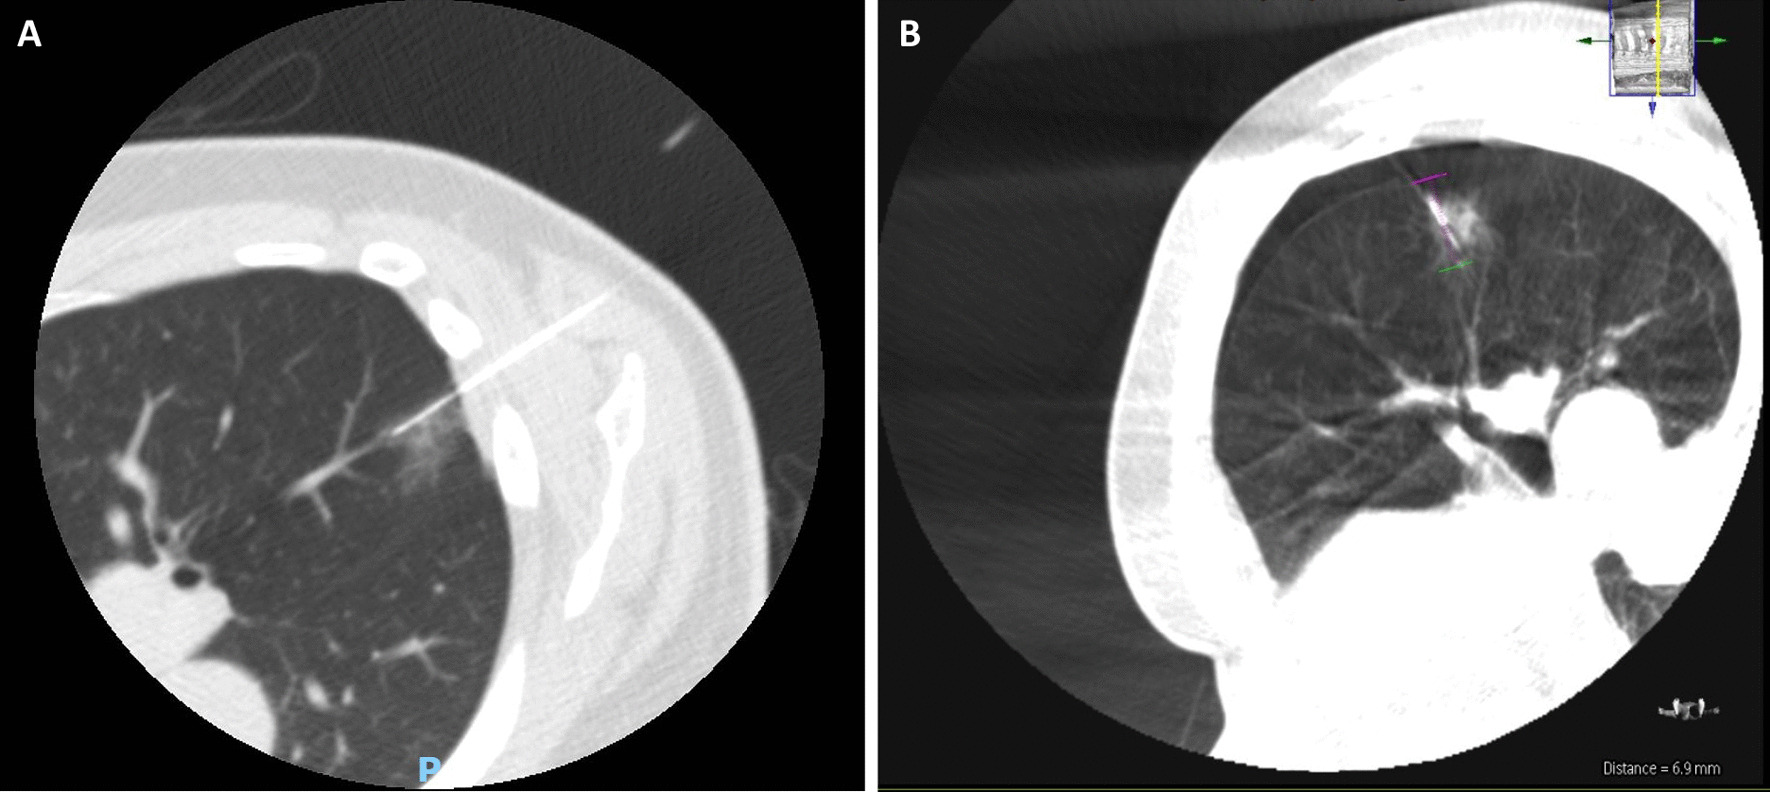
IR suite CT and intra-operative CBCT images depicting difference in resolution and visualization of pulmonary arteries. A IR suite CT with hookwire in-situ after localization of a lesion closely related to pulmonary arteries. B Intra-operative confirmatory CBCT of the same patient. Note visualization of adjacent pulmonary arteries is more challenging due to lower resolutionLesions located close to the major fissure (< 10 mm), as the higher CT resolution in the IR suite is required for better visualization of the fissure, to facilitate precise needle manipulation and reduce the risk of transpleural wire placement.Multiple lesions, as localization of the second lesion is less difficult if a pneumothorax occurs after localization of the first lesion while the patient is spontaneously breathing. On the contrary, a greater degree of positional change to the second lesion occurs in patients who develop a pneumothorax after localization of the first lesion while the patient is under positive pressure ventilation with intra-operative localization, which tends to significantly increase the size of the pneumothorax.
